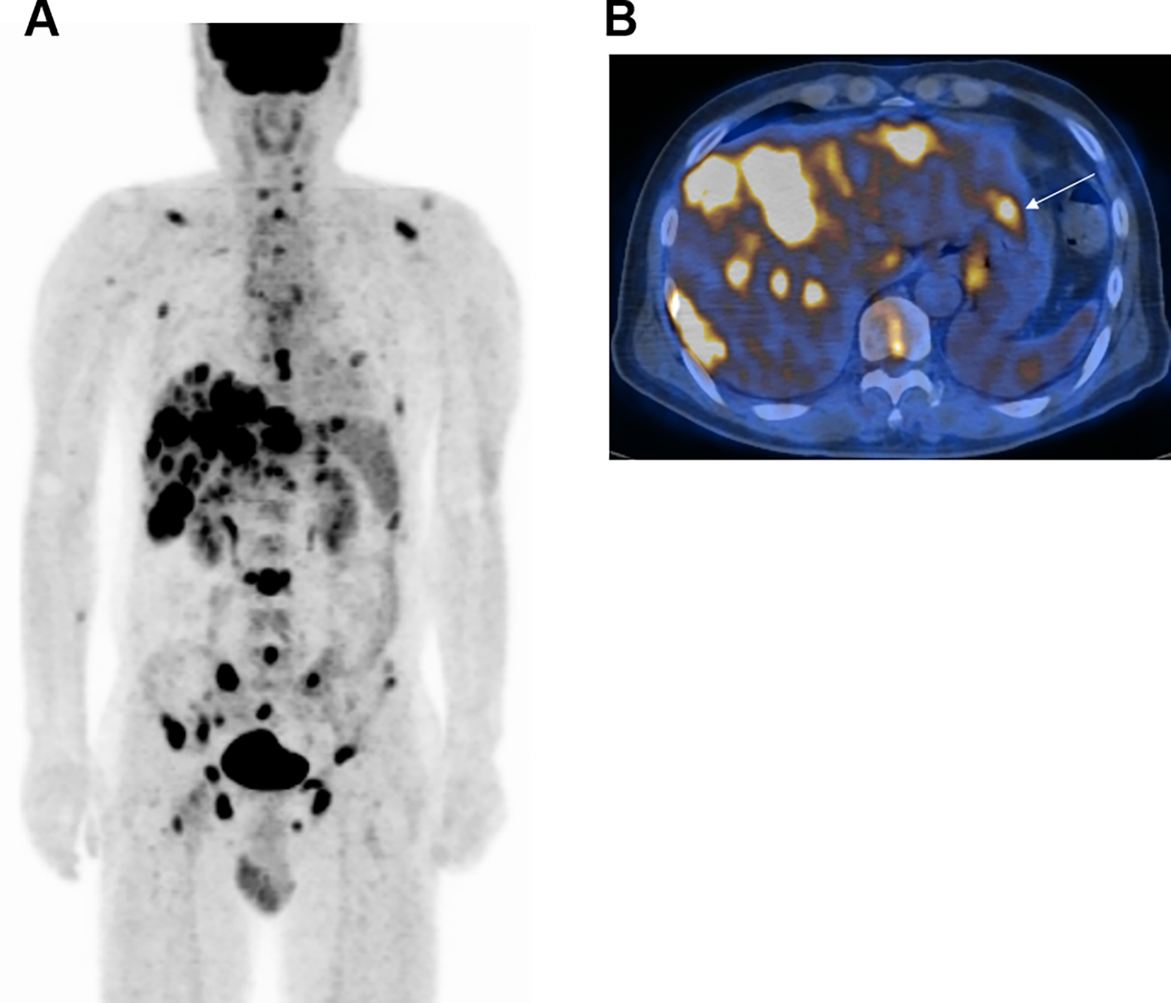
A 55-year-old male with gastric cancer identified by FDG PET/CT. (a) The maximum intensity projection image shows multiple hypermetabolic metastatic lesions in the skeleton, liver and hepatoduodenal lymph nodes. (b) A FDG lesion was detected in the stomach that was interpreted as primary gastric cancer (arrow). Endoscopy revealed a 3-cm-sized ulcerative gastric mass and biopsy confirmed adenocarcinoma of the stomach.
